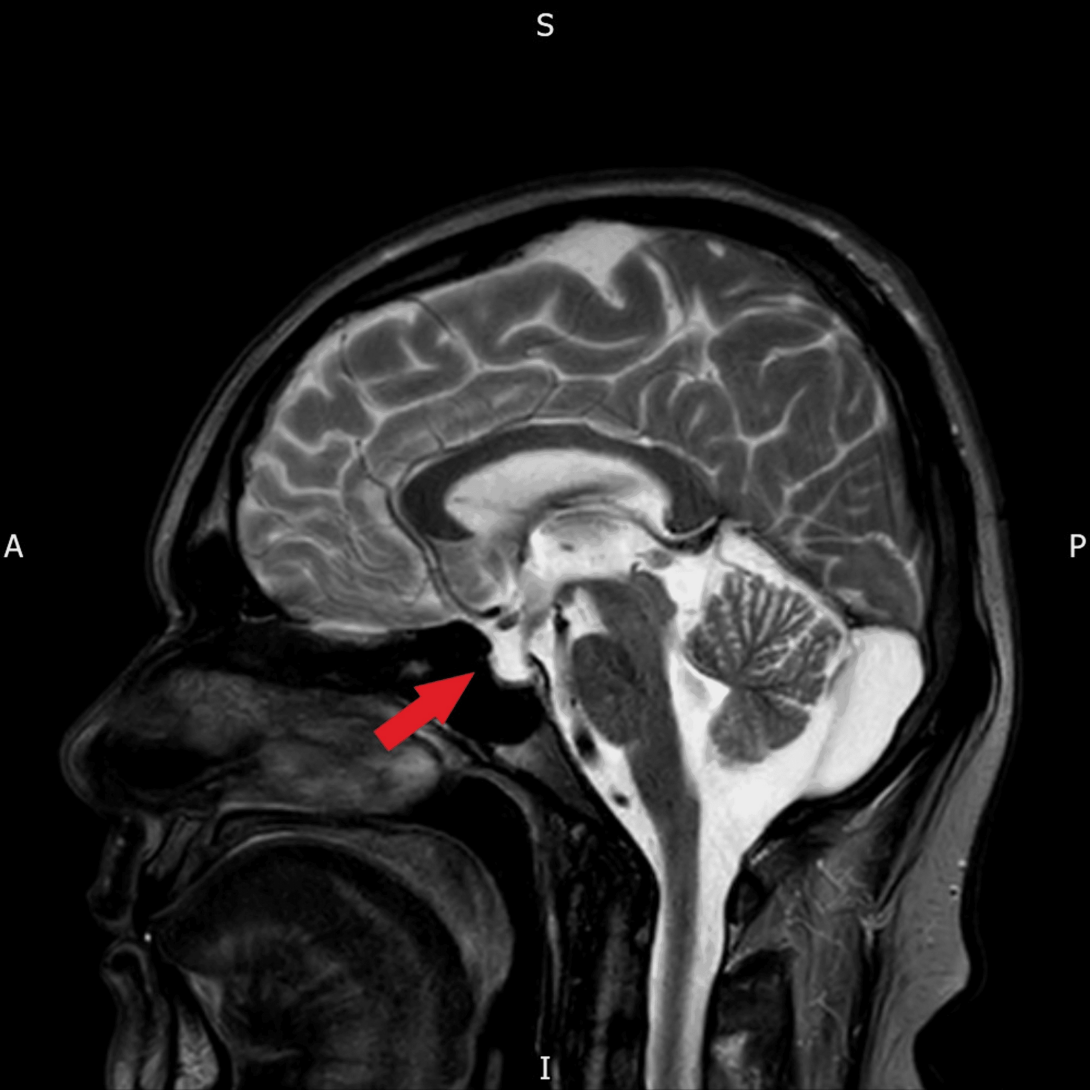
MRI of the brain showing empty sella
